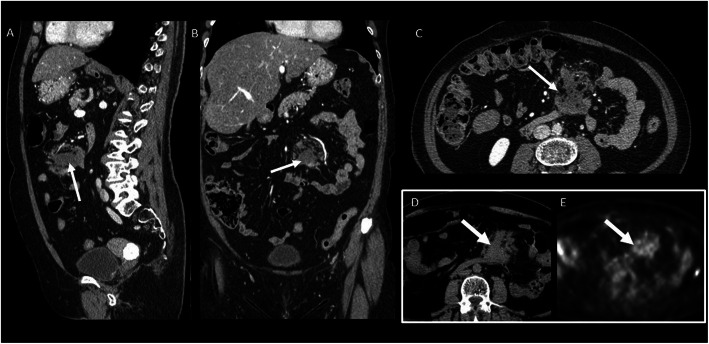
Asymptomatic patient performed a CT in order to stage a bladder cancer. Contrast-enhanced CT images ((a) sagittal, (b) coronal, and (c) axial planes) incidentally visualized ill-defined mass in the mesentery characterized by a mild contrast enhancement (white arrow). At CT-PET examination, the mass shows a mild to moderate uptake
